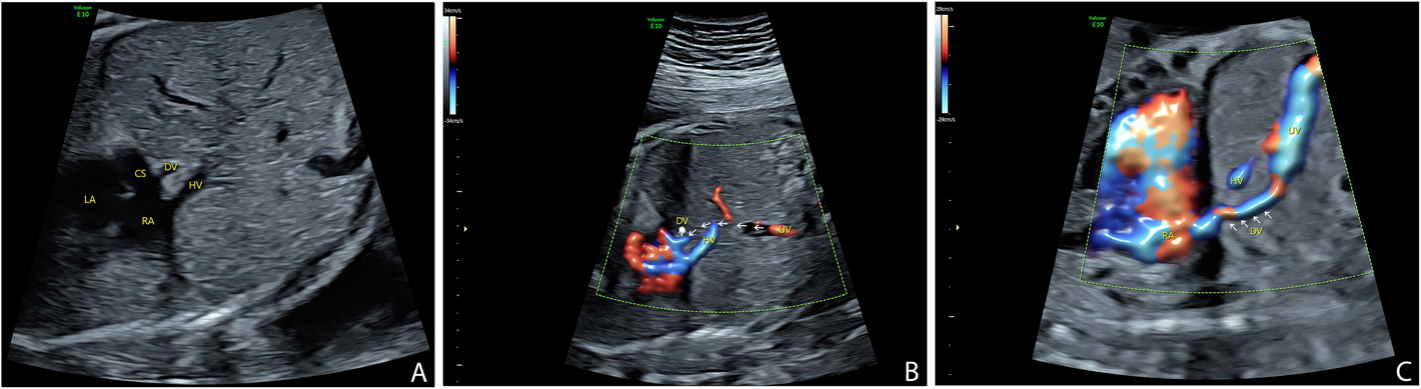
Sonograms in parasagittal view showing the aberrant course of the ductus venosus. A small vessel is visualized draining into the coronary sinus (CS) near the insertion point of inferior vena cava to the right atrium (a). This vessel (indicated by a hand mark) turns to be the ductus venosus (DV) as it connects with the umbilical vein (UV). The arrows indicate the route of UV-DV connections that cannot be shown by color imaging as the blood flow is vertical to the sound beam (b). The origin and aberrant course of DV (indicted by arrows) could be better shown in another perspective (c) HV: hepatic vein; LA: left atrium; RA: right atrium
